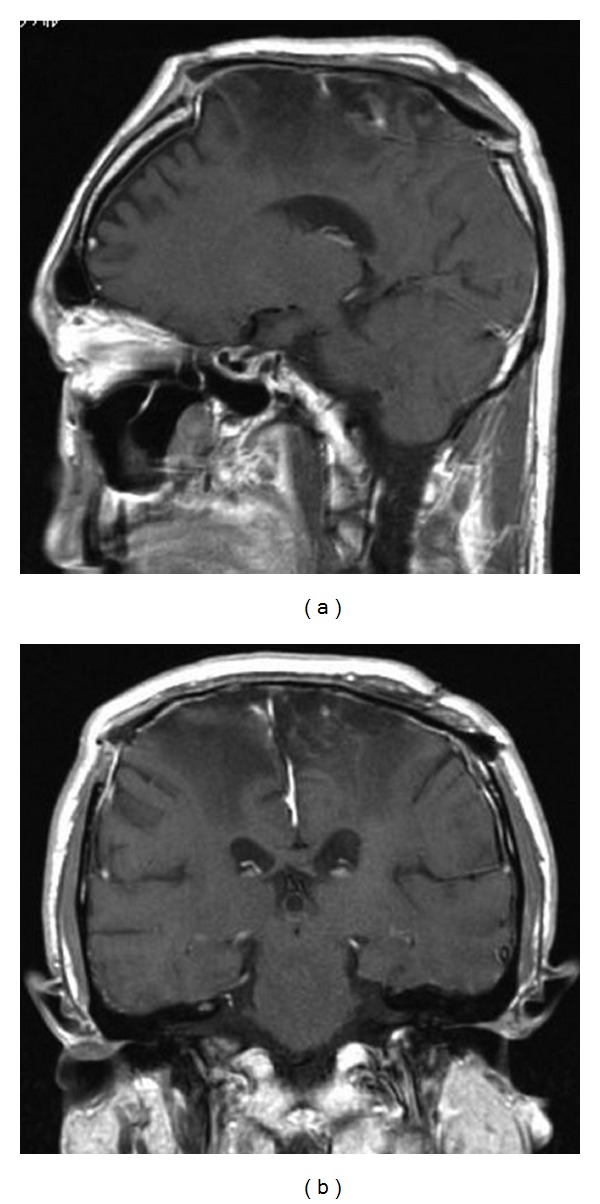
Postoperative sagittal (a) and coronal (b) T1-weighted MR images with contrast medium revealing complete removal of the tumor without recurrence.
